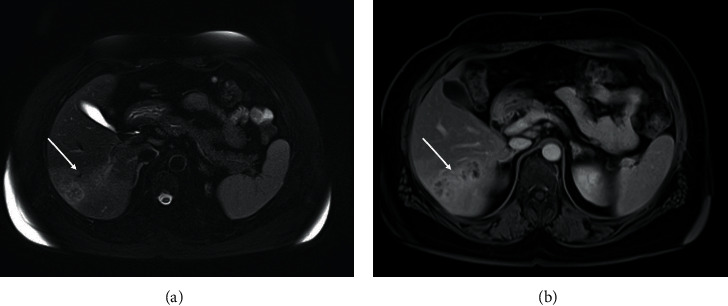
MRI scan which unveils the hepatic nodular lessions. (a) T1. (b) T2.
